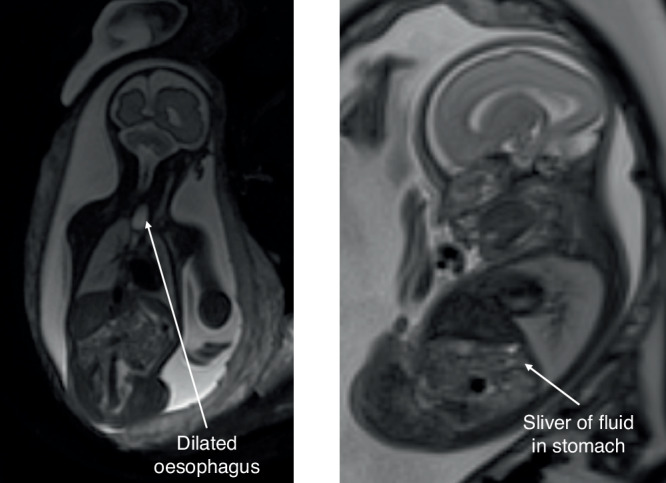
The pouch sign and a sliver of stomach fluid. Coronal T2 SSFSE MRI image of the dilated oesophagus (pouch sign) and small stomach in a 30-week fetus with TOF/OA (left) and sagittal T2 SSFSE fetal MRI image of a 25-week fetus with a sliver of fluid visible in the stomach (right).
